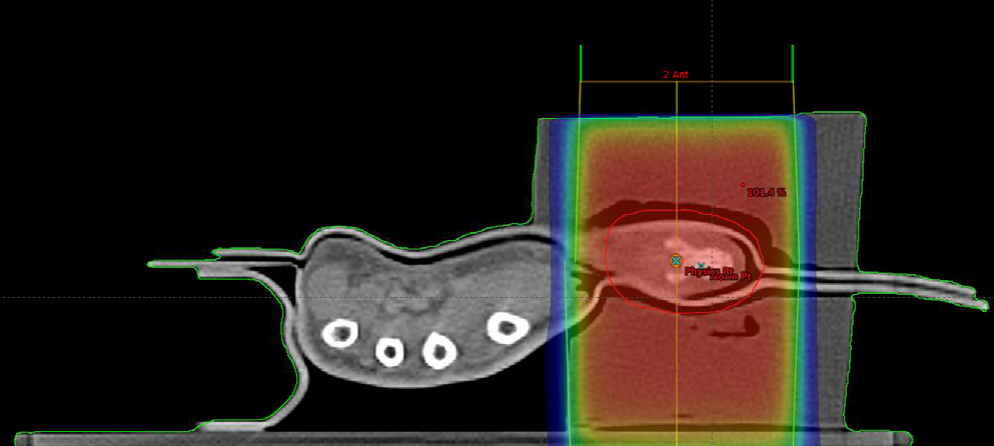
Axial plane of the planning CT showing the patients hand encased in the wax block and the dose fully covering the PTV (red outline). (For interpretation of the references to colour in this figure legend, the reader is referred to the web version of this article.)
